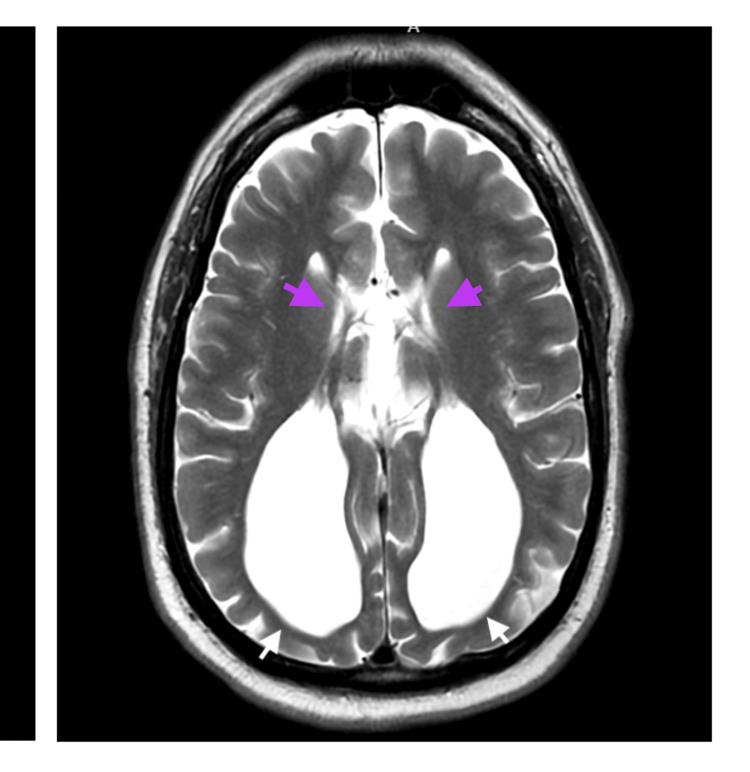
T2 propeller MRI brain axial view displaying the “racing car” sign characteristic of corpus callosum agenesis.
